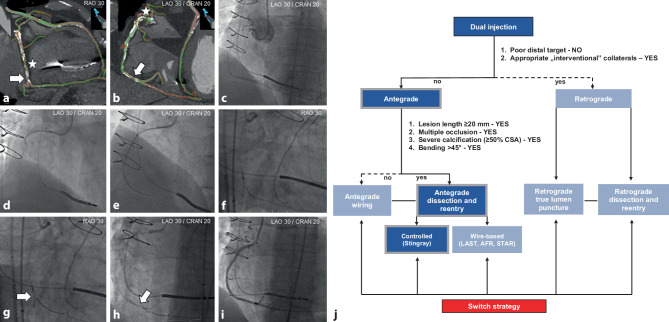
a–i Antegrade dissection and re-entry strategy of a chronically occluded right coronary artery (*RCA*). Coronary computed tomography angiography (*CTA*) multiplanar reconstructions revealing short and tapered proximal cap, long and severely calcified double occlusion site (*stars*) with bending and a relatively good distal target with multiple calcium spots. The *white arrows* indicate the favourable re-entry sites within the distal RCA (a, b). Baseline dual invasive angiography demonstrating a complex chronic total occlusion of the RCA with distal filling via collaterals from the left anterior descending coronary artery supplied by the left internal mammary artery (c). Based on the CTA-based hybrid algorithm, an antegrade dissection and re-entry strategy was selected as the primary recanalisation approach, and a knuckle Fielder XT‑A guidewire (Asahi Intecc, Nagoya, Japan) was advanced to the mid-RCA (d). After antegrade insertion of a GuideLiner (Teleflex, Wayne, PA, USA), the CrossBoss catheter (Boston Scientific, Boston, MA, USA) crossed the occlusion subintimally into the distal RCA (e). Subsequently, the CrossBoss catheter was exchanged for a Stingray balloon (Boston Scientific, Boston, MA, USA), placed adjacent to the true lumen in the distal RCA between calcium spots as indicated on CTA images (f). After multiple sticks with a Confianza Pro 12 guidewire (Asahi Intecc, Nagoya, Japan), the Gladius guidewire (Asahi Intecc, Nagoya, Japan) successfully crossed into the distal true lumen (*arrows*, g, h). Final angiographic result after implantation of multiple drug-eluting stents with restoration of antegrade thrombolysis in myocardial infarction grade 3 flow (i). The CTA-based hybrid algorithm for the presented case (j). *AFR* antegrade fenestration and re-entry, *CRAN* cranial, *LAO* left anterior oblique, *LAST* limited antegrade subintimal tracking, *RAO* right anterior oblique, *STAR* subintimal tracking and re-entry
